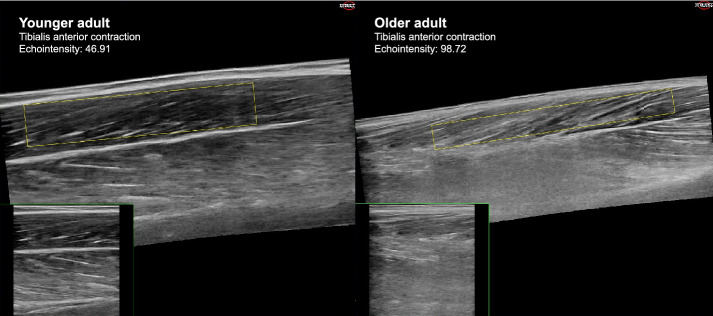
Example ultrasound scan of the m. tibialis anterior in contraction of a younger (left) and older adult (right). The yellow rectangles demonstrate the regions of interest selected for the echo intensity analysis. The mean pixel intensity of the regions of interested are displayed on the image
